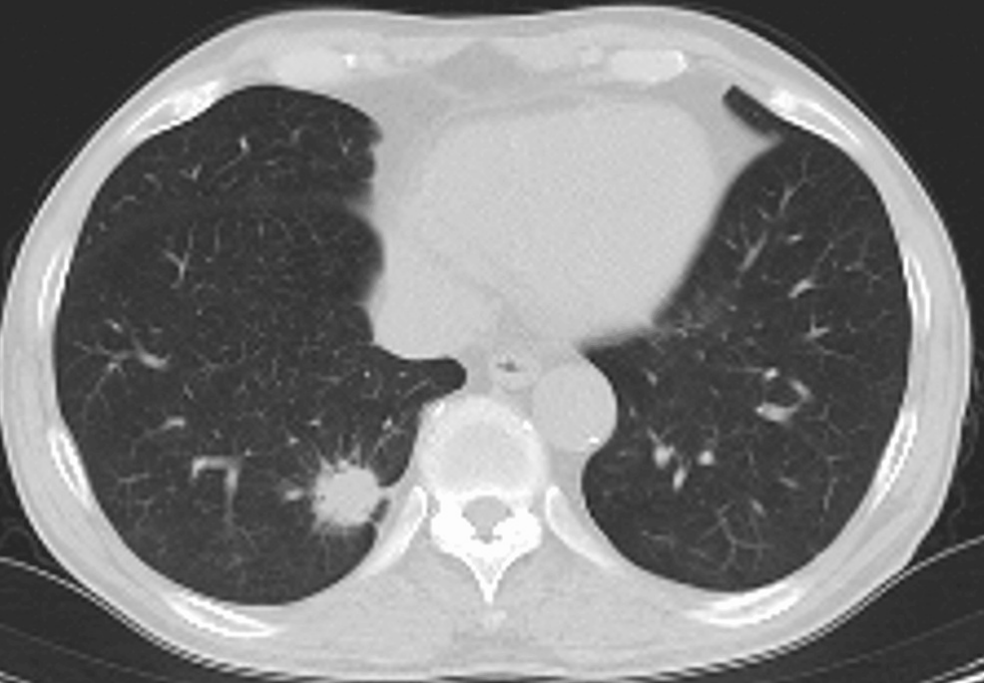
Plain chest CT imaging of the patient. Plain chest CT imaging revealed an irregular nodular shadow with spiculation in the right lower lobe.
